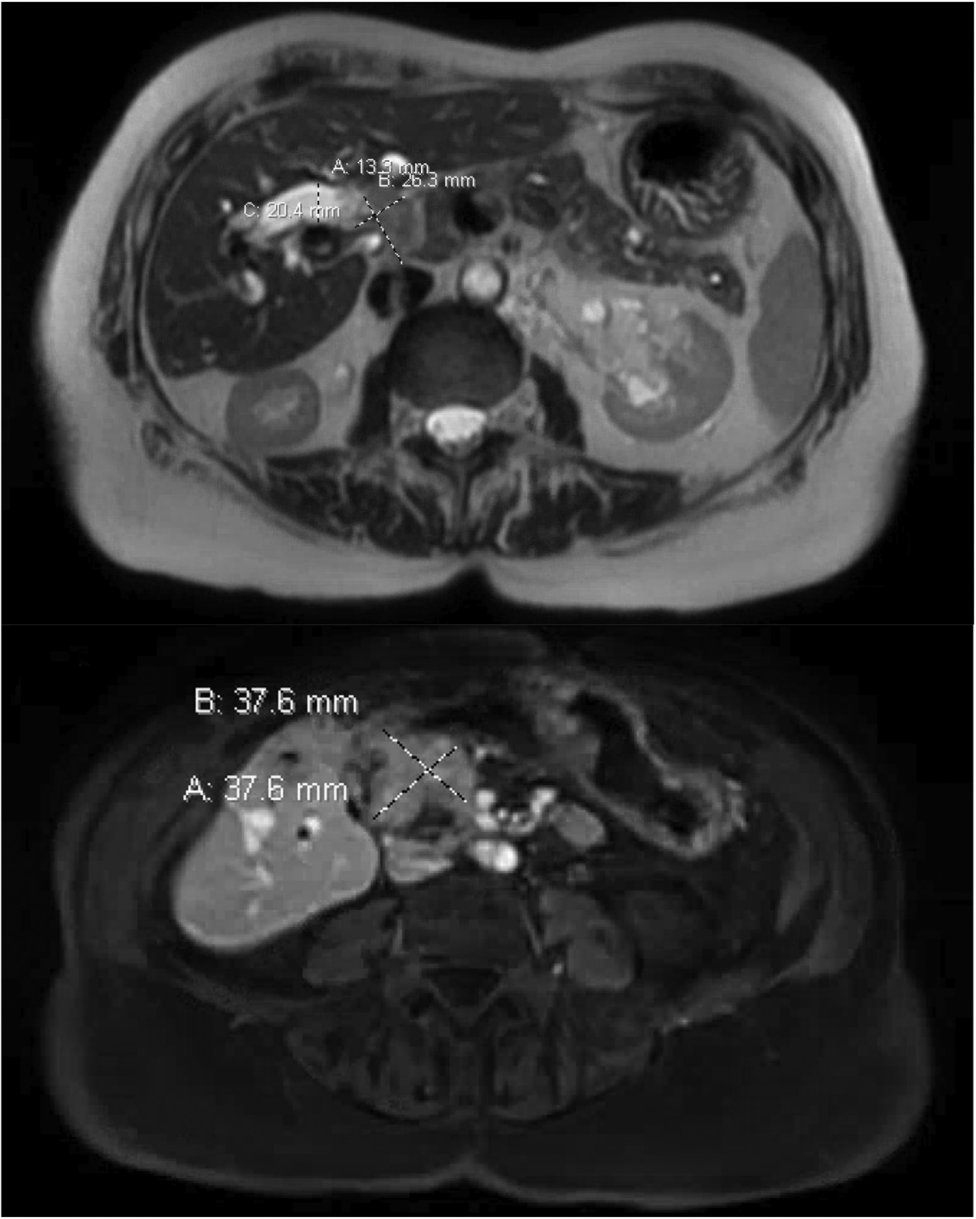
Pretreatment magnetic resonance imaging (2 months before radiation therapy start date) demonstrating the ill-defined exophytic lesion measuring 3.8 × 3.8 cm in the papilla.
